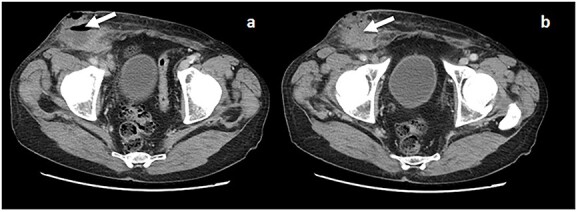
(a and b) CT scan with evidence of a fluid collection in the right inguinal area (arrow) and an apparent recurrent hernia.
